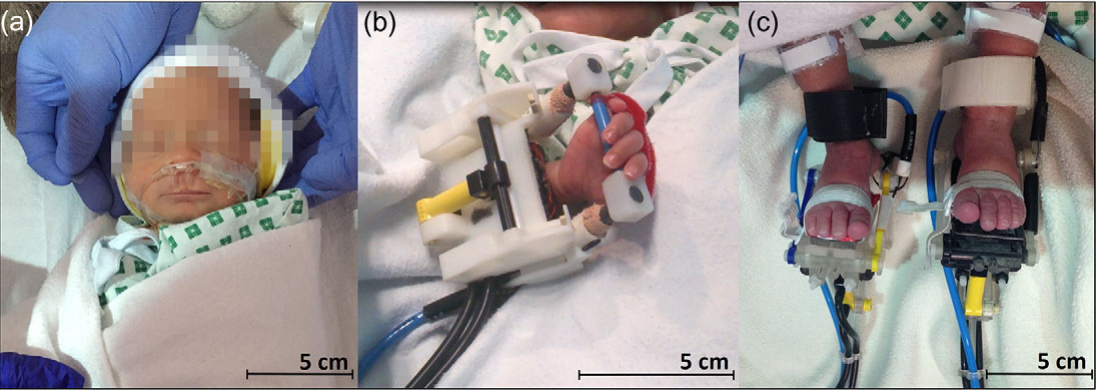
MRI-compatible automated devices used for sensory stimulation. A soft puff of air was delivered to the mouth via inverted clinical nasal cannula (*a*), while pressurized air was used to actuate the yellow piston in the robotic devices resulting in controlled flexion and extension movements of the wrist (*b*) and ankle (*c*).
